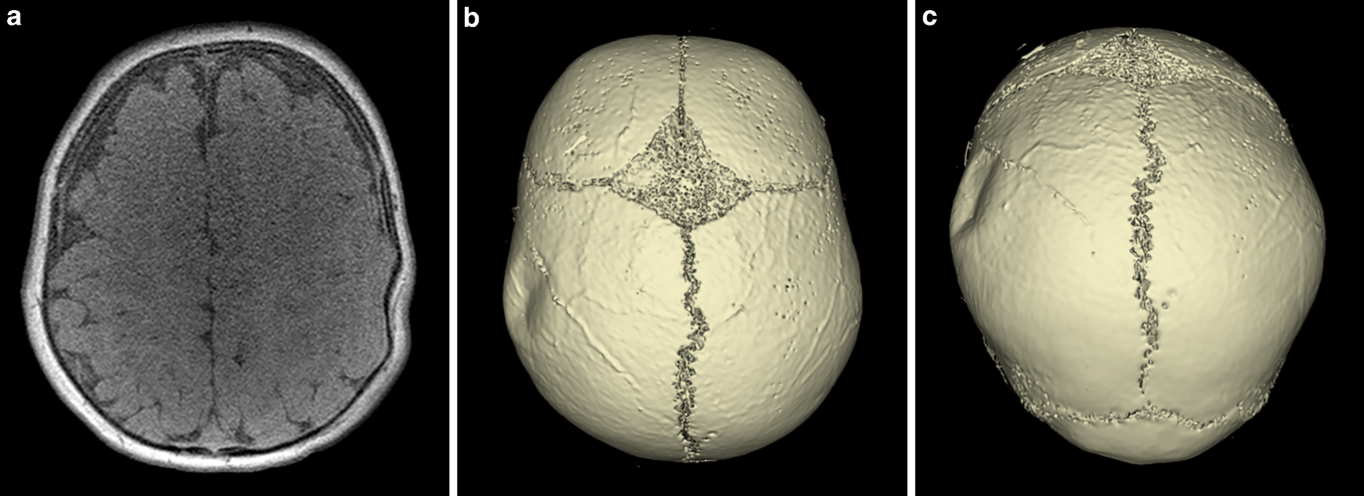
Axially acquired 3D T1-weighted spoiled gradient echo sequence (a) with which 3D reconstructions were performed (b and c) of the skull of a 6-month-old male patient with a ping pong fracture of the left parietal bone. It should be noted that the unfused sutures can clearly be depicted on the 3D reconstructions
